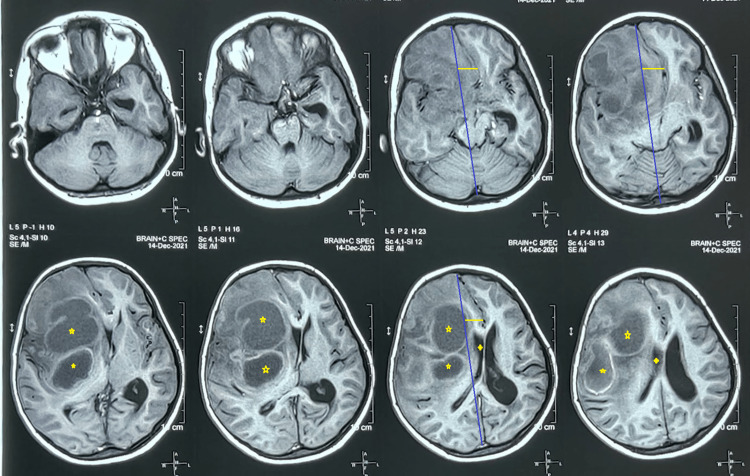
T1 MRI The images show multiple variable-sized well-defined thick-walled lesions (five-point star) in the right frontal lobe and gangliocapsular region; perifocal vasogenic edema and significant mass effect (depicted by straight lines) with partial effacement (four-point star) of the right lateral and third ventricles are also noted MRI: magnetic resonance imaging
